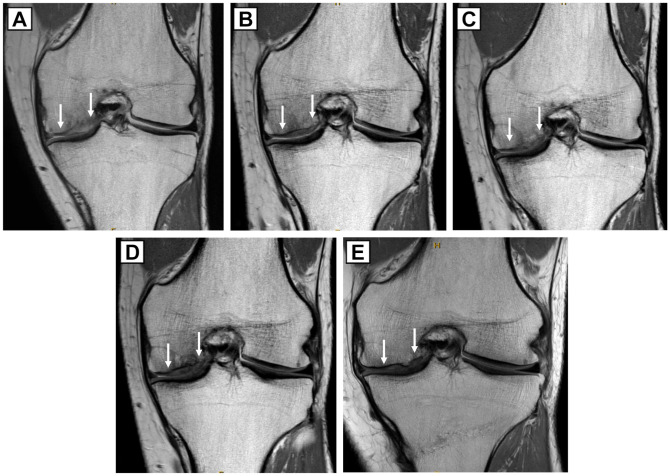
Proton density fast spin echo magnetic resonance imaging of a matrix-induced autologous chondrocyte implantation (MACI) graft (between white arrows) to the medial femoral condyle of the same patient at (A) 3 months, (B) 1 year, (C) 2 years, (D) 5 years, and (E) 16 years after surgery (after undergoing high tibial osteotomy at 5.5 years after MACI).
